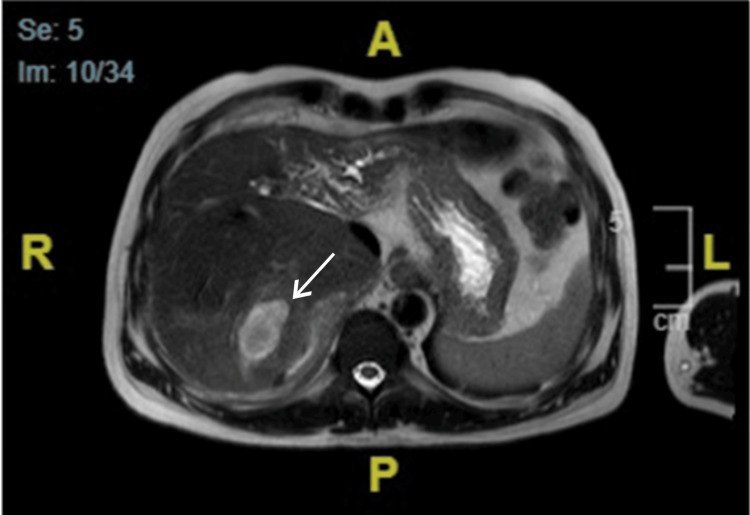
Axial magnetic resonance imaging of the liver. An ill-defined mass is seen in the right hepatic lobe showing high signal intensity in T2.
